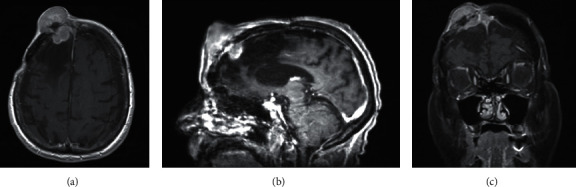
Preoperative MRI. Representative images from T1-weighted postcontrast MRI in the (a) axial, (b) sagittal, and (c) coronal orientations demonstrate a bilobed homogeneously enhancing lesion of the right frontal scalp with intracranial extension.
